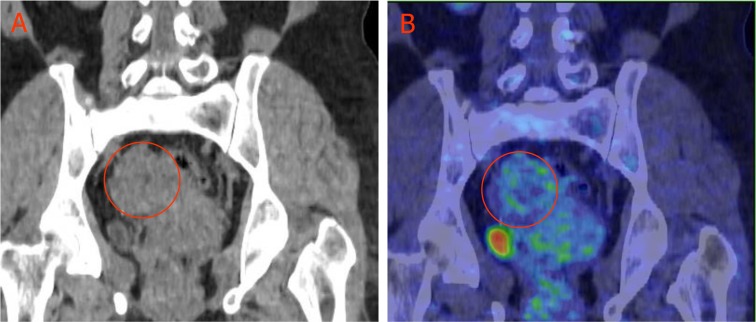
Low ^18^F-FDG uptake in ovary granulosa cell tumor A 54-yr-old female with pathologically confirmed granulosa cell tumor of the right ovary (circled), ^18^F-FDG accumulation in the tumor is low. A: CT imaging; B: ^18^F-FDG PET/CT overlay obtained 60 min after 5 mCi (185MBq) ^18^F-FDG intravenous injection.
